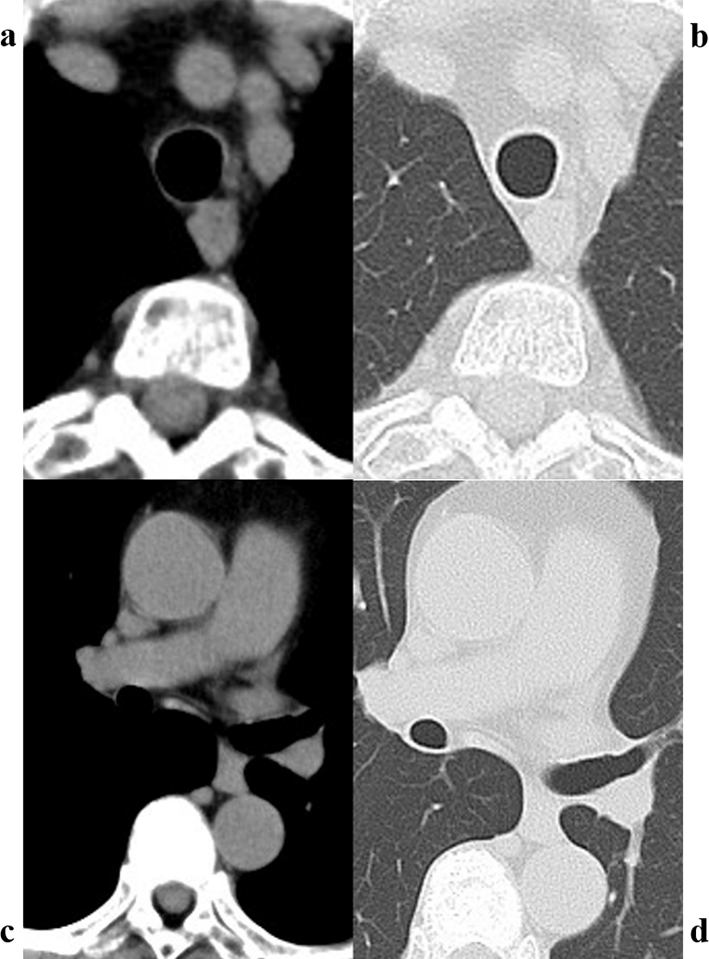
Chest computed tomography (CT) images at the level of the trachea (a, b) and the left main bronchus (c, d) after 6 months of treatment show considerable improvement in the lesions
